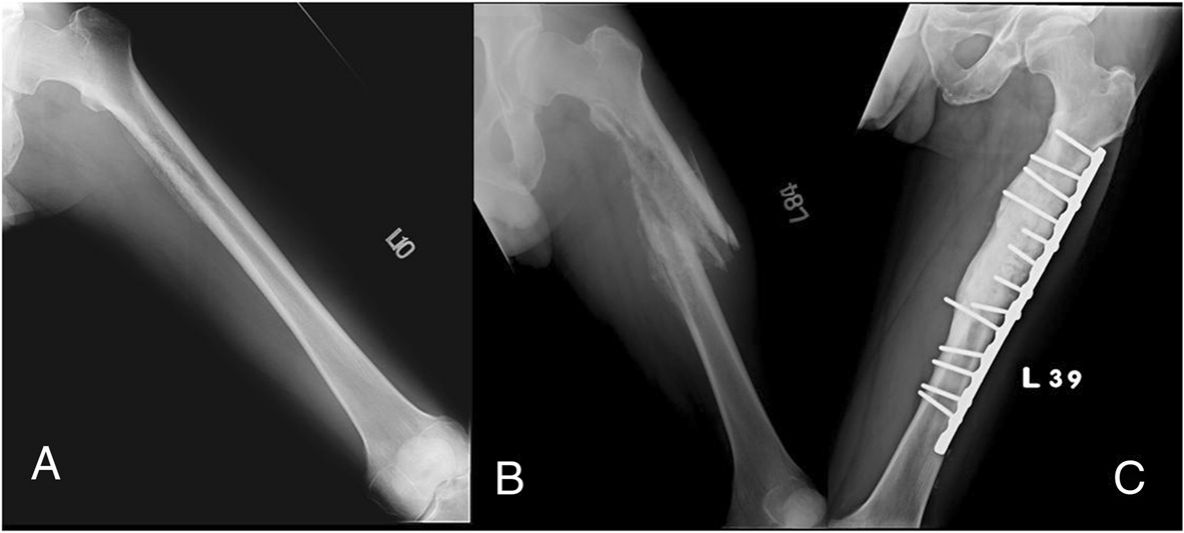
A 57-year-old man who complained of nocturnal thigh pain for four months. (A) Preoperative plain film showing a permeative osteolytic lesion and cortical disruption with laminated periosteal reaction. (B) Plain film taken one month after debridement surgery, showing a pathological fracture with severe periosteal reaction and cortex thinning. (C) Two years after open reduction and internal fixation (ORIF) and multiple debridement surgeries: antibiotic impregnated-bone cement at medial cortex and bony union without recurrent signs.
